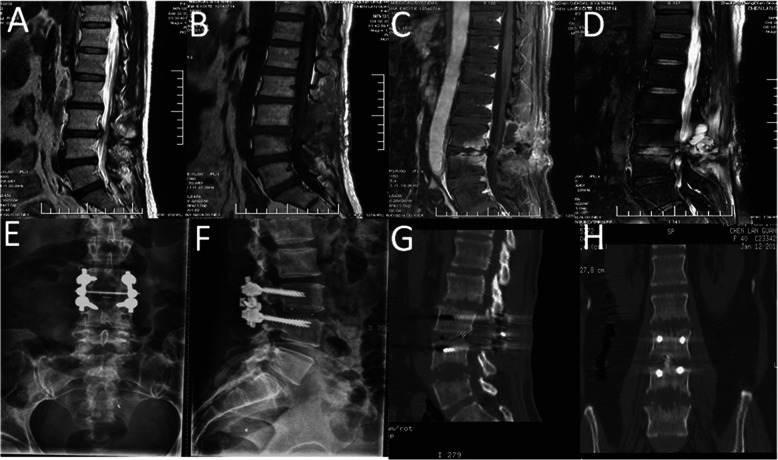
Sequential radiologic findings in a previously healthy 40-year-old man (case 1). Prior to primary lumbar discectomy, sagittal T2-weighted MRI showed a herniated disk at L3/4 (a). The original single-level infection was detected by T1-weighted MRI, approximately 2 weeks after primary discectomy (b). One month later, T1- and T2-weighted MRI showed the infection had progressed to L3/4 (c) and (d). Lesion debridement + bone graft fusion + pedicle screw fixation were performed in another hospital(e) and (f). CT showed that the infection had progressed to the adjacent L2/3 and L4/5 levels, with endplate destruction 2 months after fixation surgery(g) and (h)
